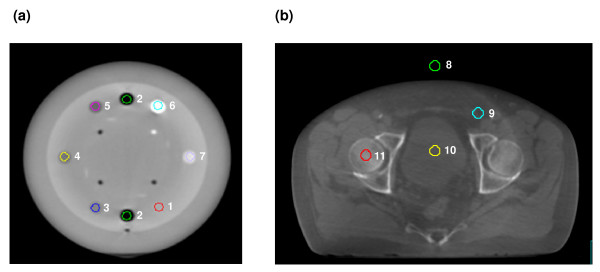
(a) Axial slice of CATPhan^® ^geometry acquired with the CBCT system and volumes of interest for each material insert: 1 Acrylic, 2 Air, 3 Polystyrene, 4 LDPE, PMP, 6 Teflon^®^, 7 Delrin^®^. (b) Example of volume definition for a pelvis patient: 8 air, 9 fat, 10 fluid, 11 femoral head.
